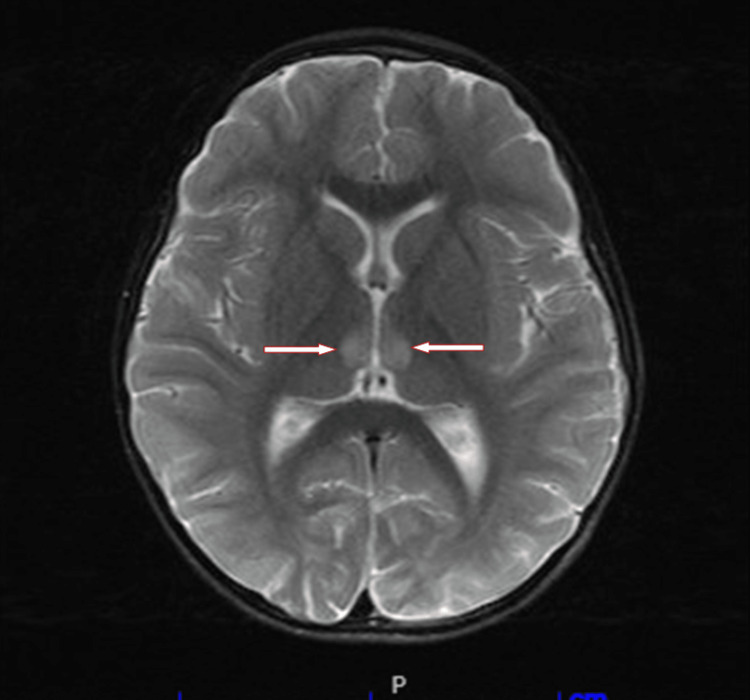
Brain MRI showing abnormal hyperintense lesion in the mammillary bodies.
